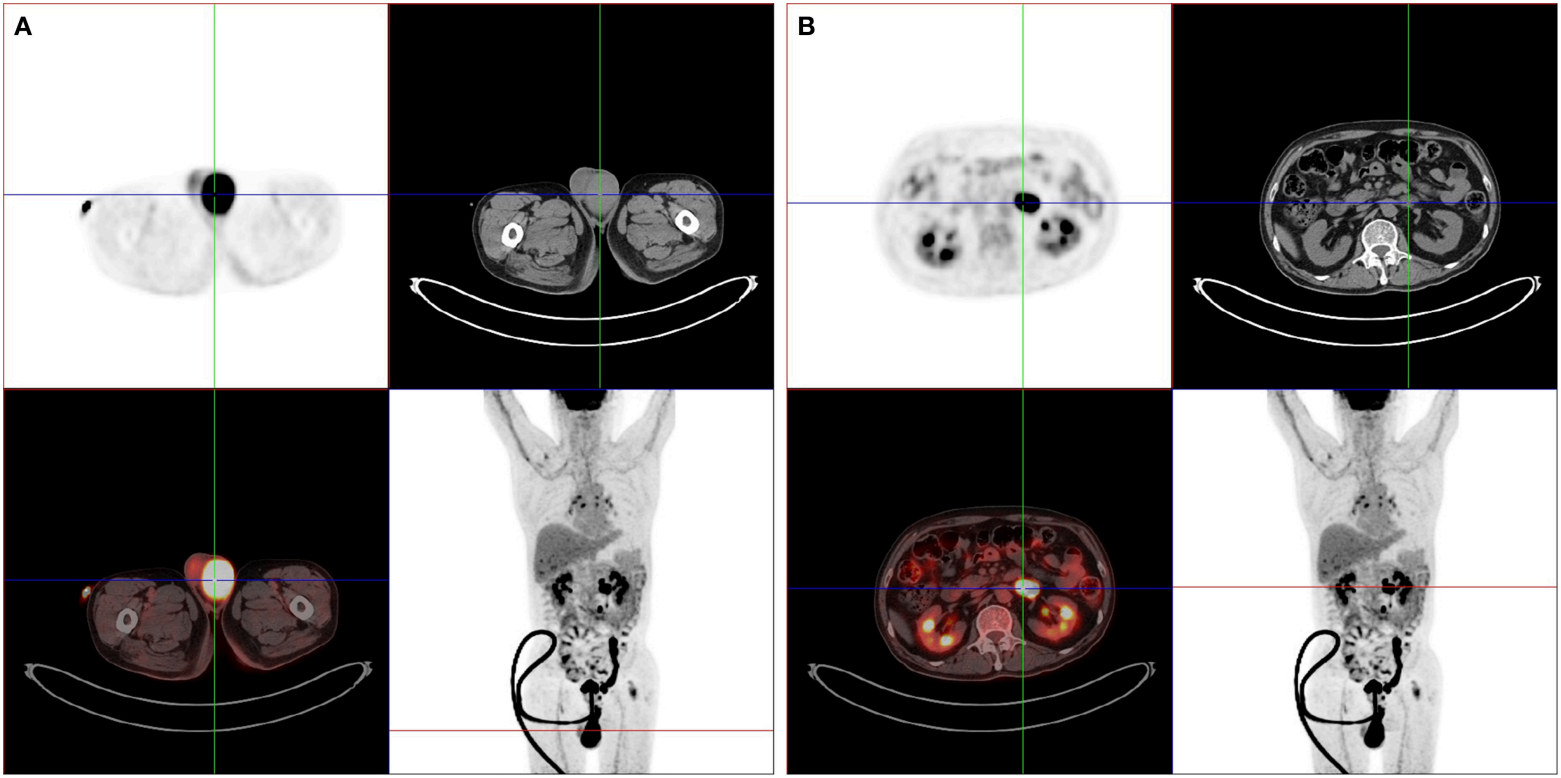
Whole-body PET/CT findings. PET/CT imagings showed abnormal hypermetabolic lesions in the left testicle (A) and in multiple abdominal and retroperitoneal lymph nodes (B).
